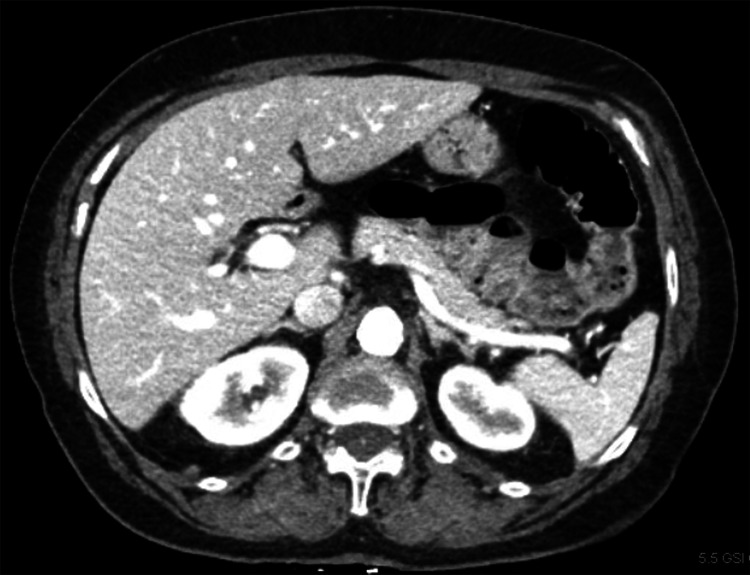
Spiral abdomen and pelvic CT showing complete resolution of the gastrohepatic ligament lymph node.
